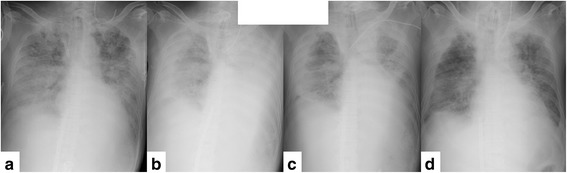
Trends in X-ray imaging. a Day 11. b Day 22. c Day 30. d Day 34. Note the drastic improvement on day 30
